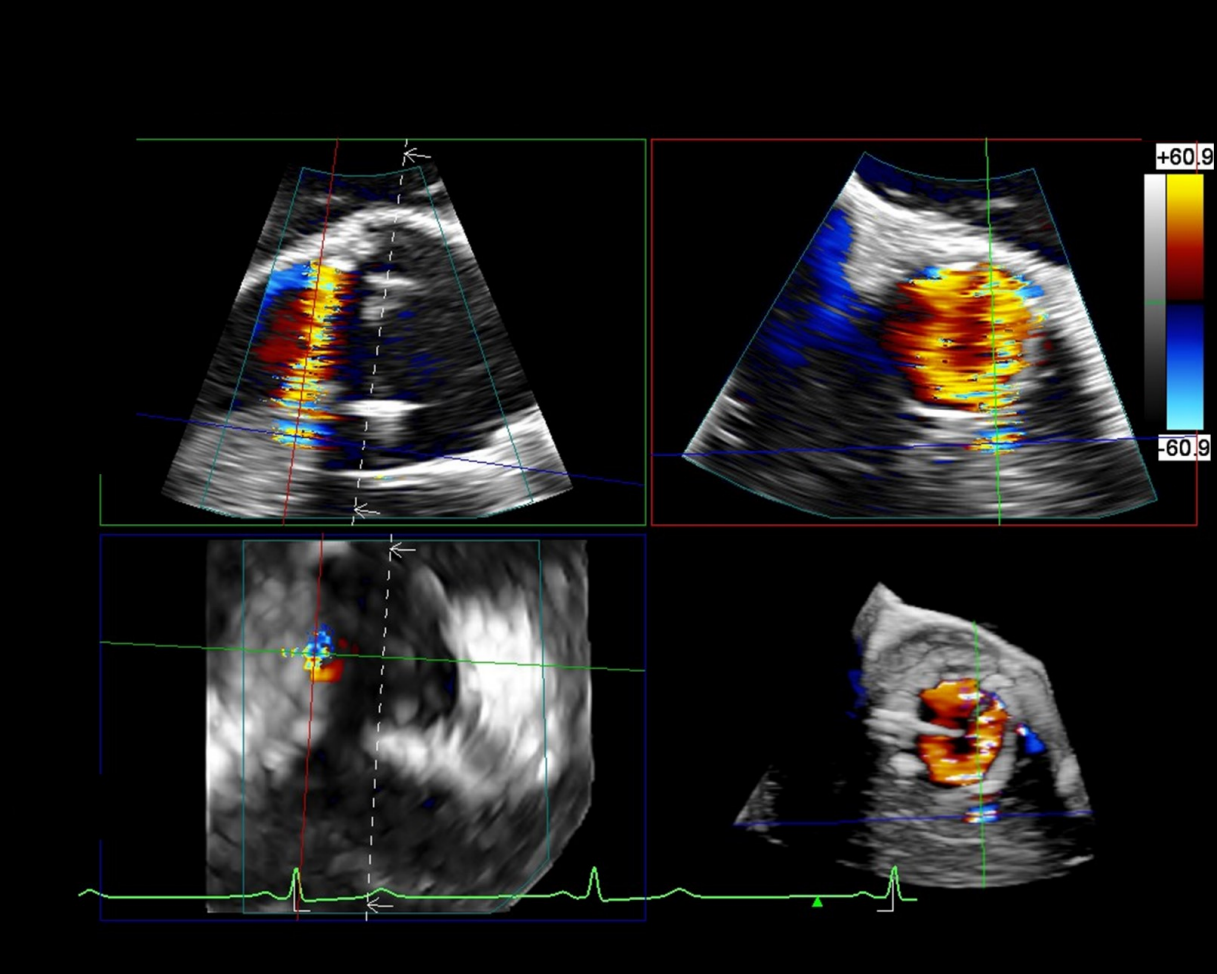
Transesophageal echo with paravalvular leak. Patient with #27
